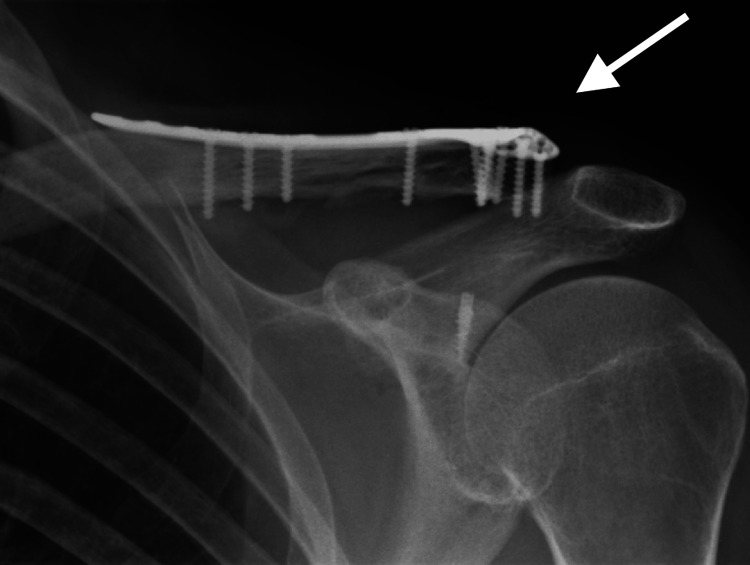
Radiographs at eight weeks.
